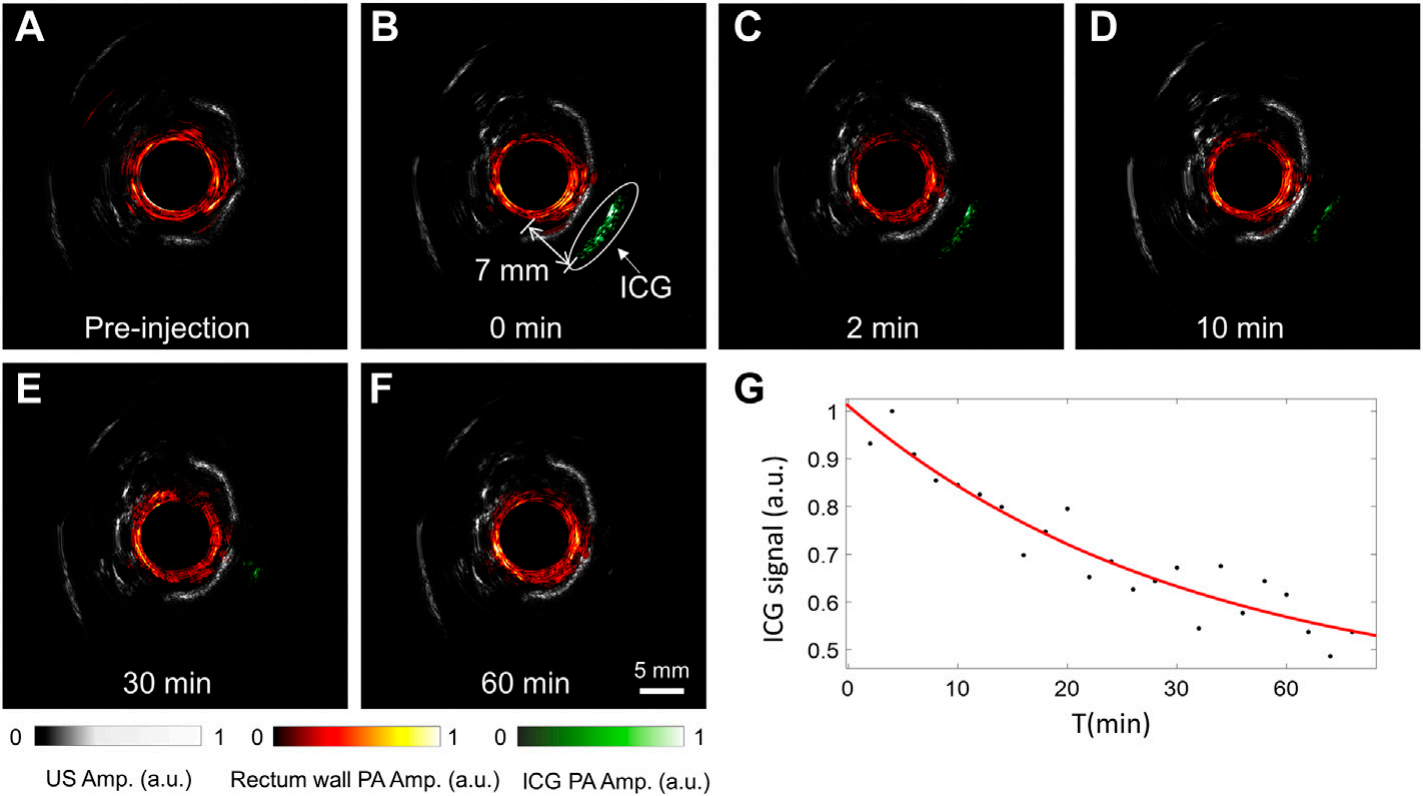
Experimental results for tracking the injected ICG near the rabbit rectum. (A–F) Comparison of photoacoustic and ultrasound images of the rabbit rectum before (A) and after (B–F) ICG injection, with the image acquisition time indicated. (G) The change of the peak ICG signal with time. The drug injection time was set to 0.
